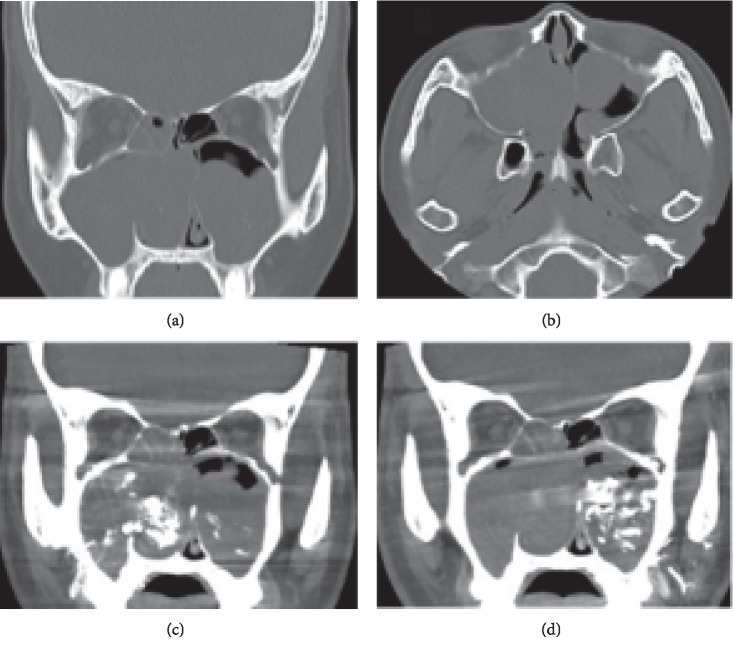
Noncontrast computed tomography and computed tomography angiography images. Preoperative (a) coronal and (b) axial noncontrast computed tomography shows a soft tissue mass filled the bilateral nasal cavities and maxillary sinuses without bony destruction. Computed tomography angiography shows that (c) the main feeder of right-side tumor was greater palatine artery and (d) the other side was infraorbital artery.
